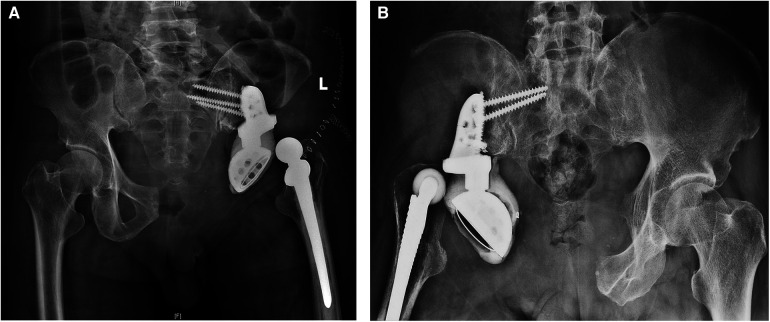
Postoperative complications after prosthetic hemipelvic reconstruction. (A,B) Postoperative x-ray showing dislocation of the hip after prosthetic hemipelvic reconstruction in two patients.
